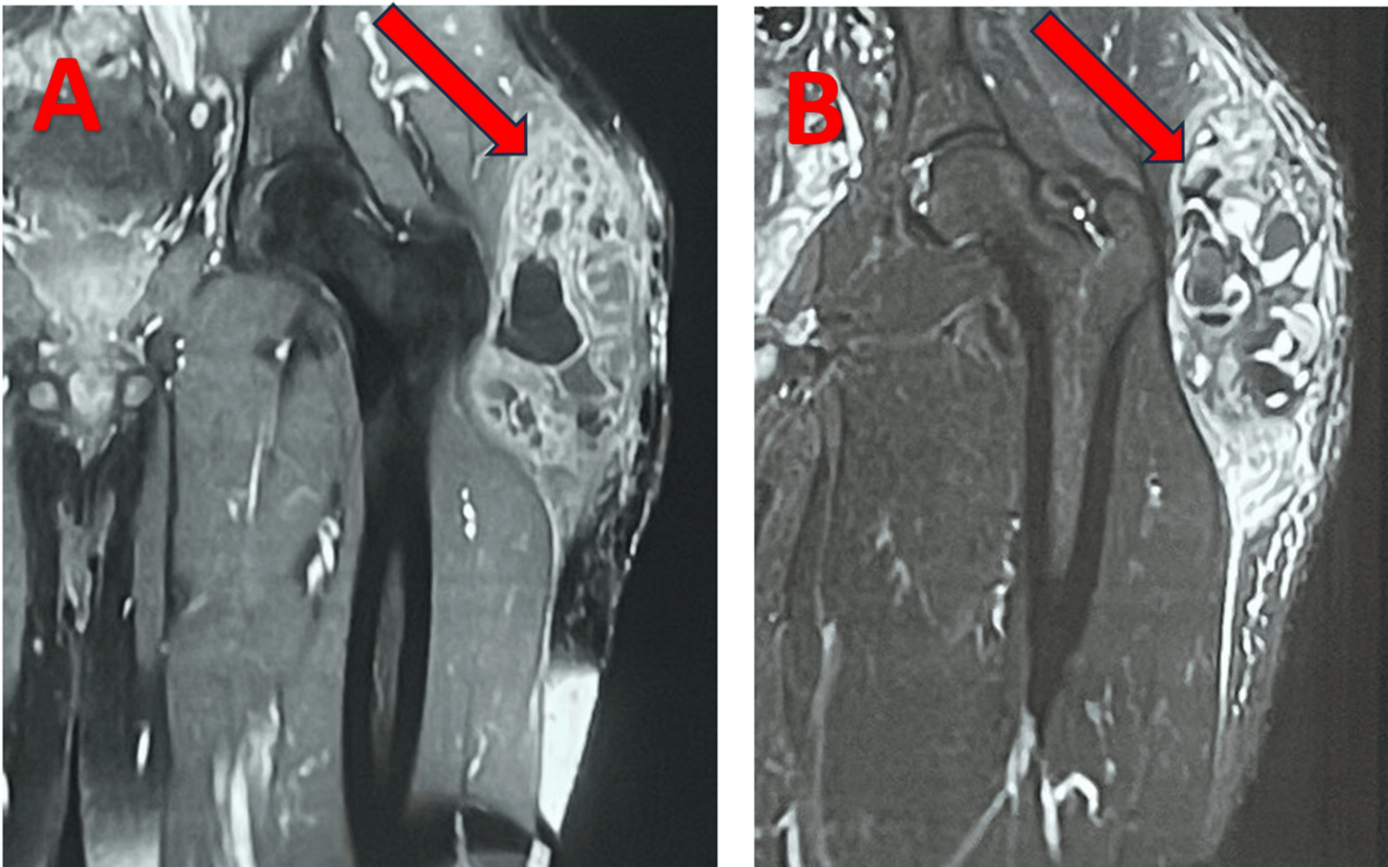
MRI findings of the left hip: A: Non-contrast MRI (coronal view) showing a collection with multiple calcifications over the left greater trochanter (arrow). B: Contrast-enhanced MRI (coronal view) showing peripheral enhancement of the collection (arrow).
